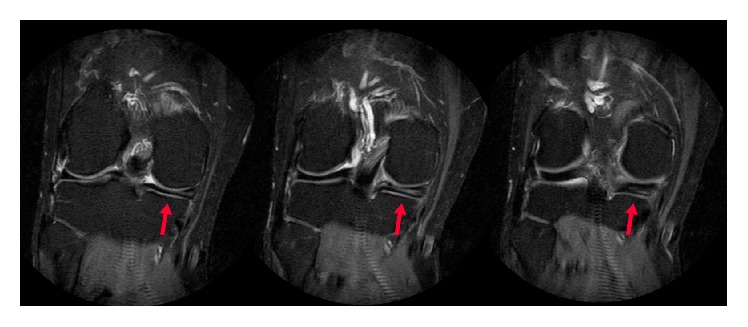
Coronal Proton Density (PD) fat suppression (FS) magnetic resonance imaging showing the chondral flap with an appearance similar to that of a torn medial meniscus (arrow). No underlying bone edema is seen.
